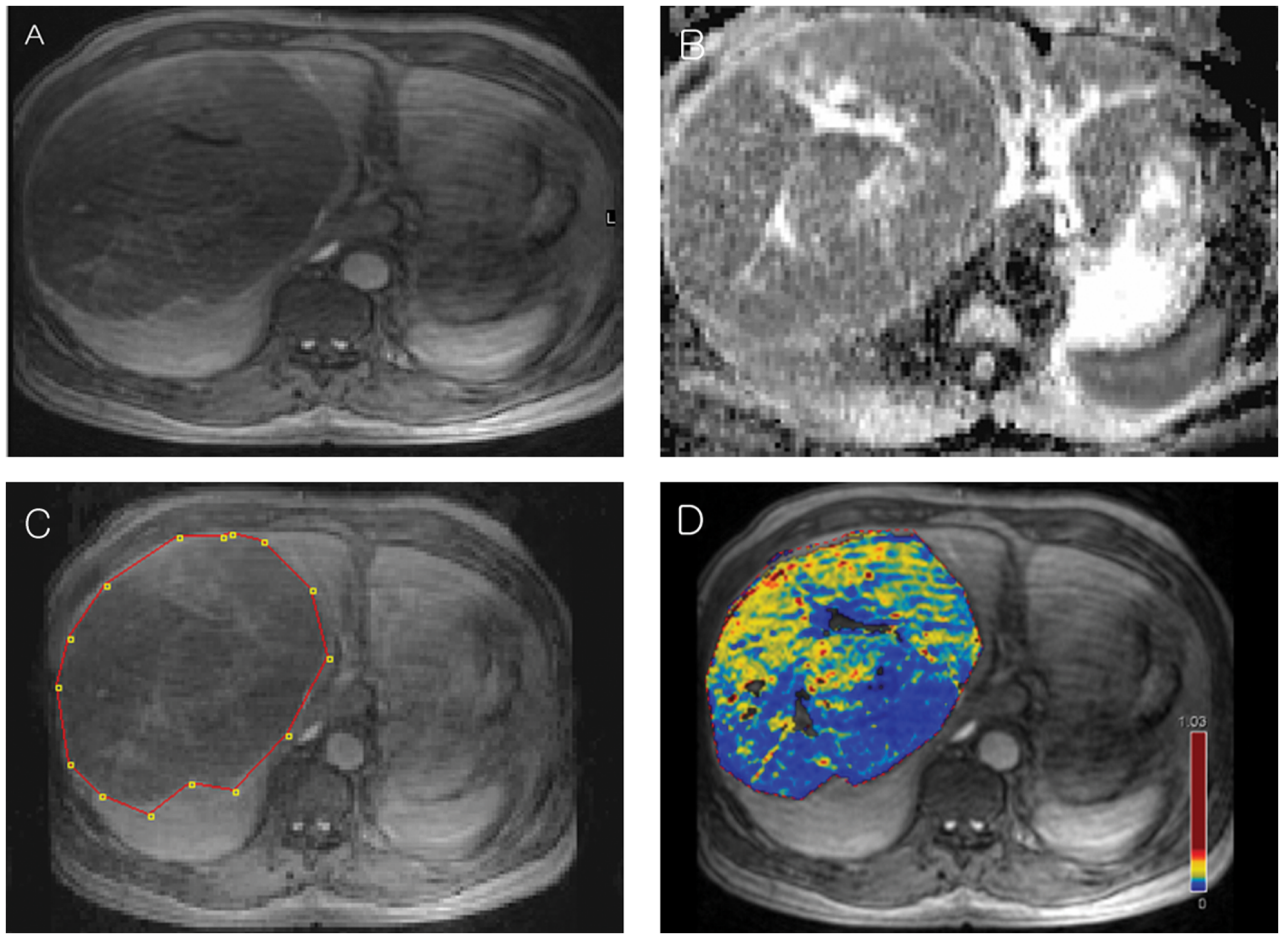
Advanced HCC in the Rt. lobe is well differentiated from normal liver parenchyma on delayed phase of DCE MRI(A), 7∼8 min after contrast injection. After ADC map (B) is coregistered to delayed phase of DCE MRI(A), ROI(red dotted line) was drawn along the tumor border on the coregistered image(C) and ADC value was calculated. K^trans^ color coded map(D) is also achieved based on the delayed phase of DCE MRI.
